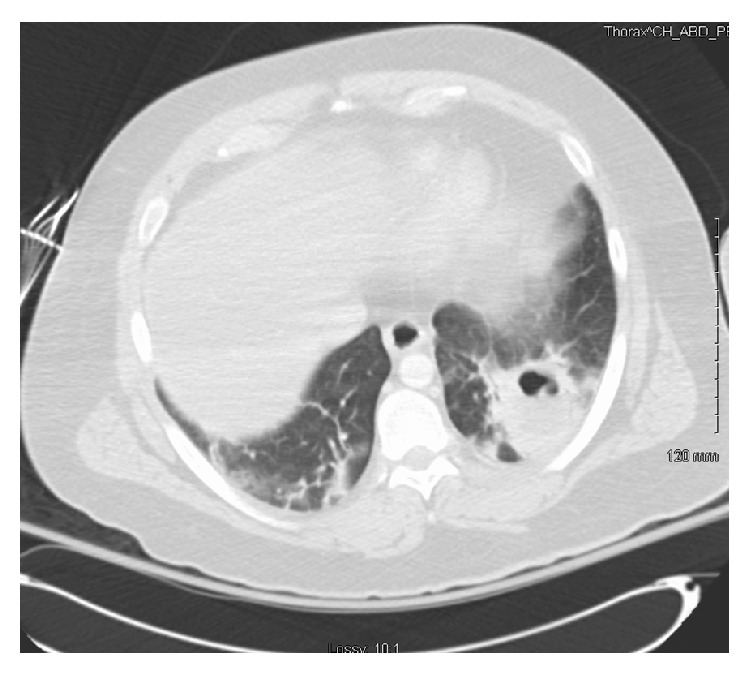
CT of the chest where left lower lobe cavitary lesion is evident.
